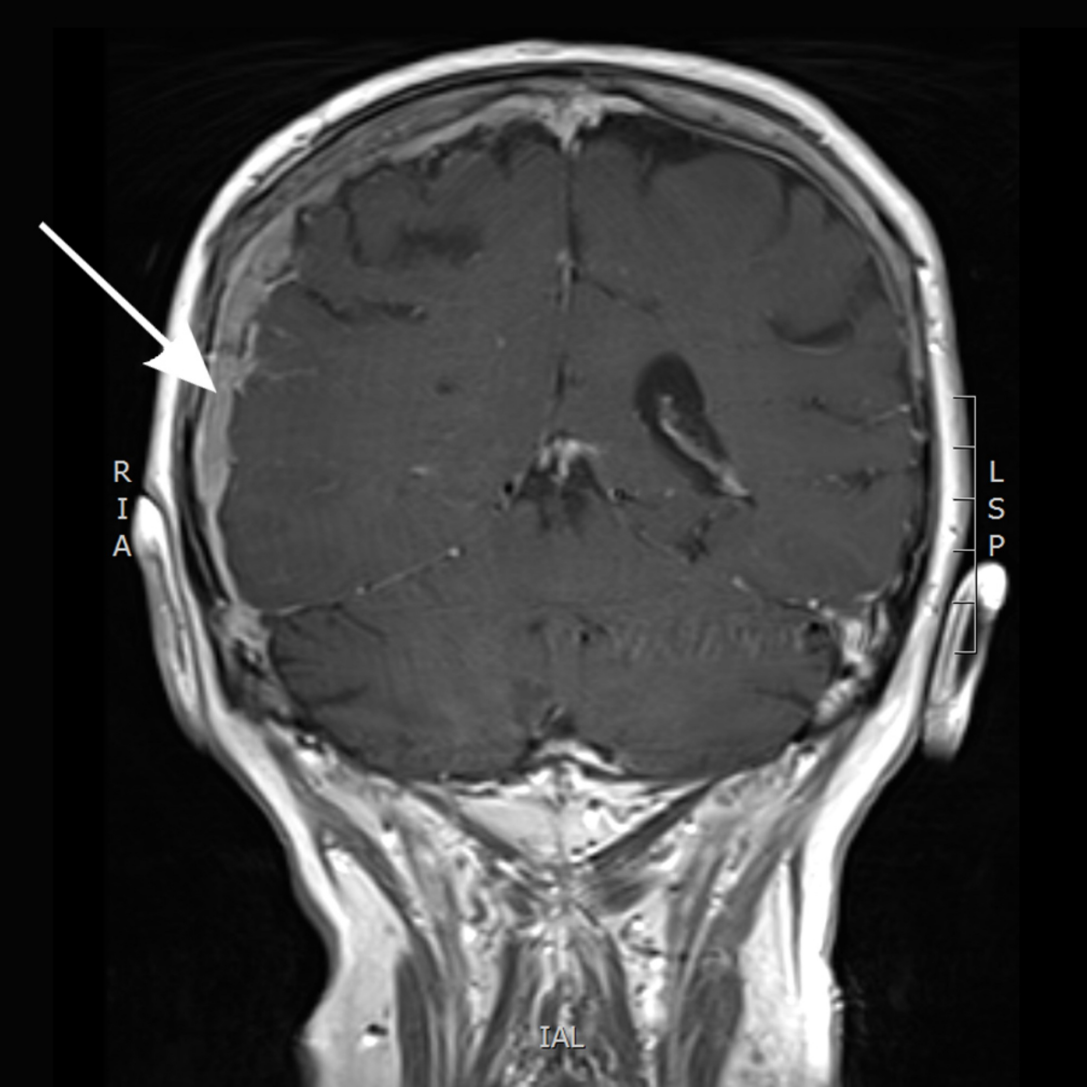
Magnetic resonance imaging (MRI) with gadolinium contrast showing pachymeningeal metastatic disease overlying the right cerebral convexity
